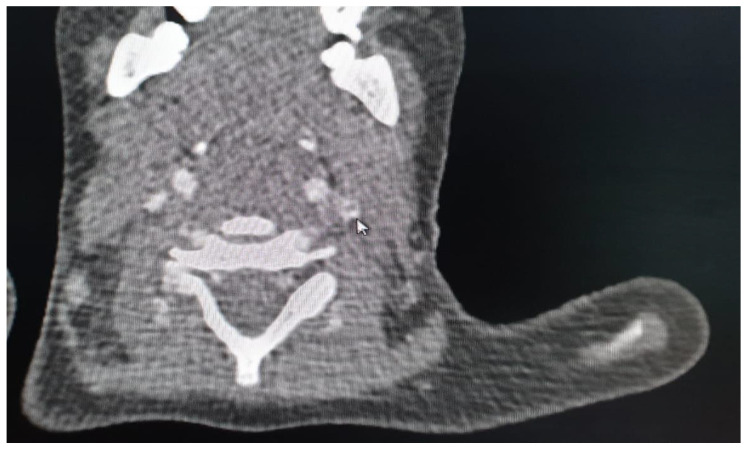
CT scan, left IJV thrombosis. (white arrow).
